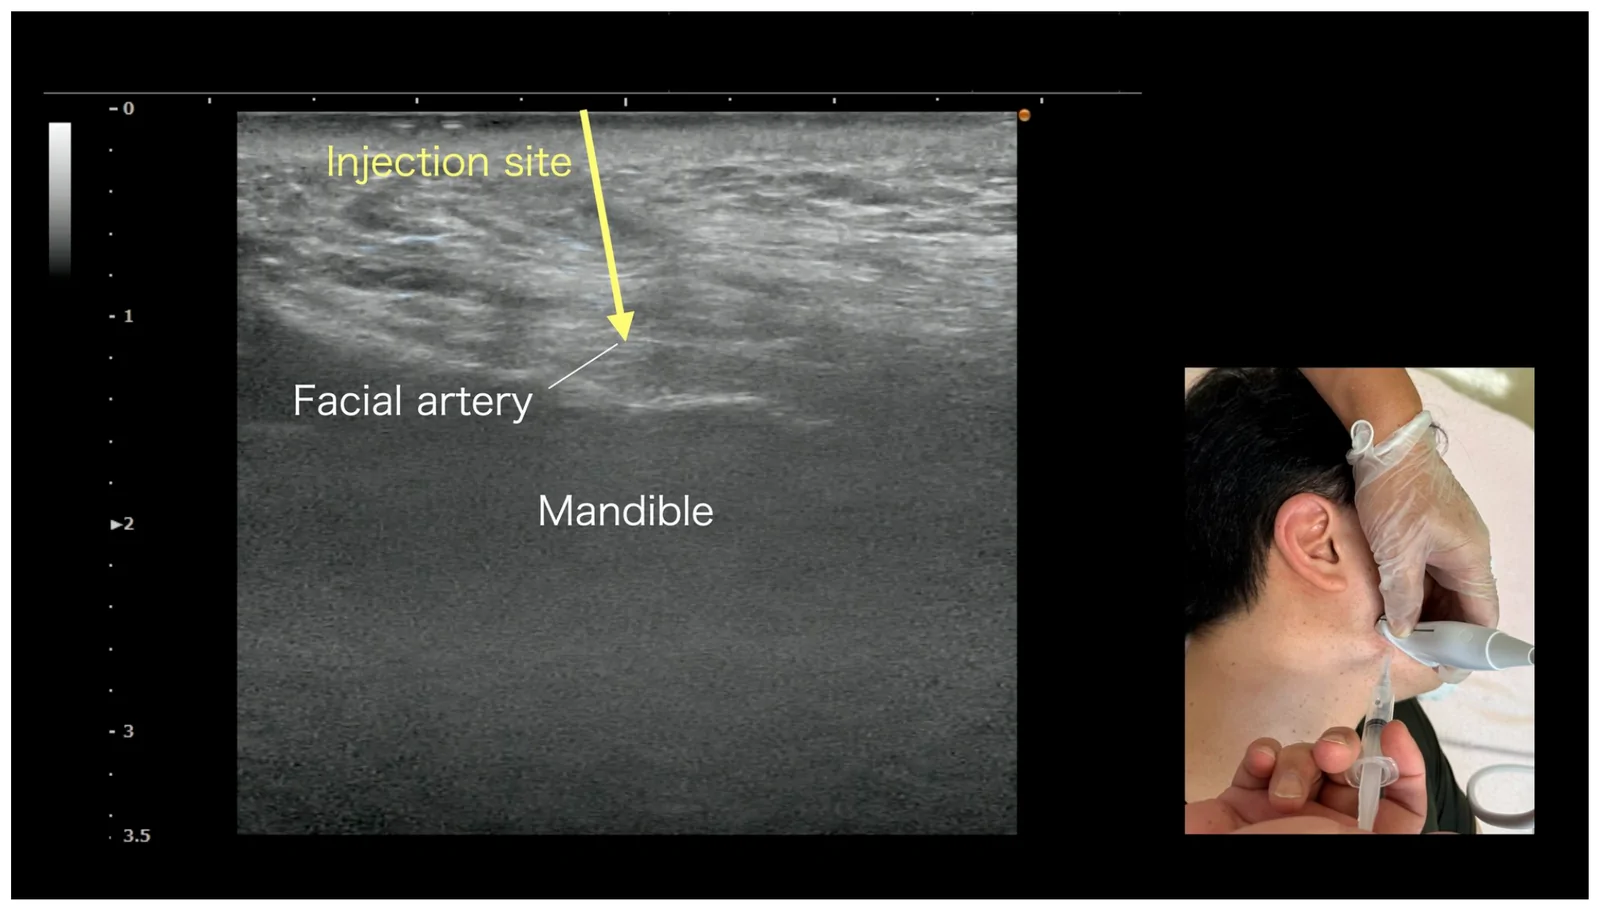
Perivascular fascia of the facial artery US-FHR (POINT 10). Ultrasound image: facial artery and mandible are identified. After confirmation of the artery with color Doppler, the yellow arrow (injection site) indicates the fascia lateral to the arterial sheath. A small volume is injected. Color Doppler identification of the artery is mandatory for safety; Doppler is turned off during the release. The inset (bottom right) shows the patient position and probe placement.
